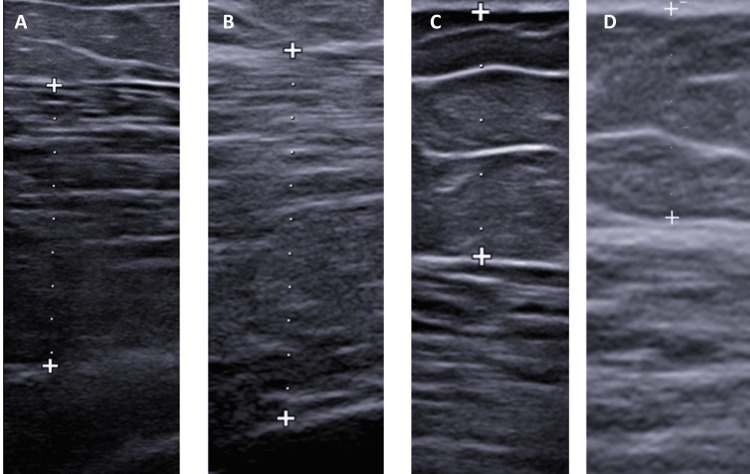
Diagnostic ultrasound images at the baseline and at the end of the protocol Diagnostic ultrasound images of the left gluteus maximus of the patient treated with both FMS and Sonic Shaper at the baseline (A) and at the end of the protocol (B); the plus signs and the white dots mark the diameter of the gluteus maximus. Diagnostic ultrasound images of the subcutaneous adipose tissue over the left gluteus maximus of the patient treated with both FMS and Sonic Shaper at the baseline (C) and at the end of the protocol (D); the plus signs and the white dots mark the thickness of the subcutaneous adipose tissue FMS: functional magnetic stimulation
